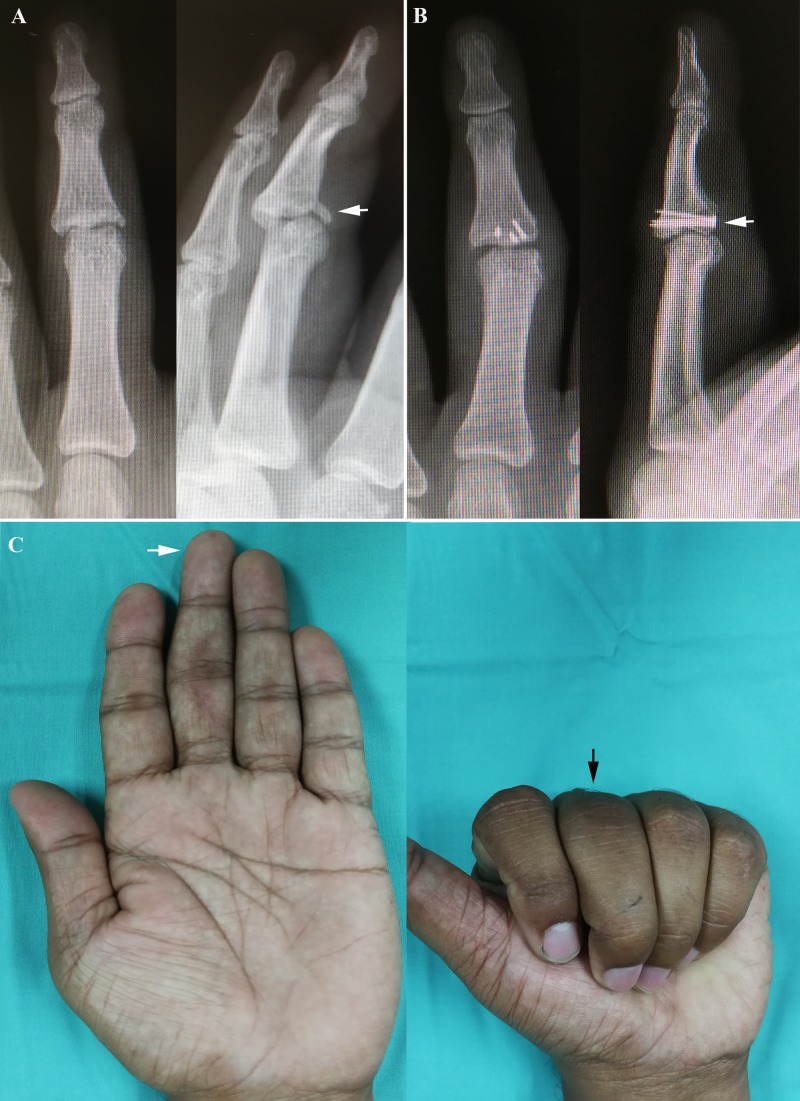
Case Report One (A) Preoperative radiographs showed fracture base of middle phalanx left middle finger with dorsal subluxation of proximal interphalangeal joint (white arrow). (B) Postoperative radiographs revealed graft union with no joint subluxation (white arrow). (C) After 12 months, good functional range of motion of the left middle finger in extension (white arrow) and flexion (black arrow).
